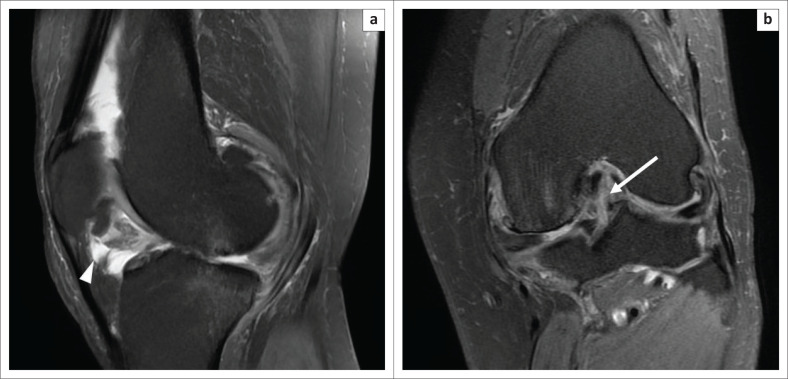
Magnetic resonance imaging of an infrapatellar plica tear in a 60-year-old female with a history of anterior knee pain: PDFS oblique sagittal image (a) shows a hyperintense fluid collection along the entire course of infrapatellar plica (arrow head) with oedema in the surrounding Hoffa’s fat pad. Oblique coronal PDFS (b) shows that the patient also had a high-grade partial thickness tear of the middle and upper thirds of both bundles of the anterior cruciate ligament (arrow).
